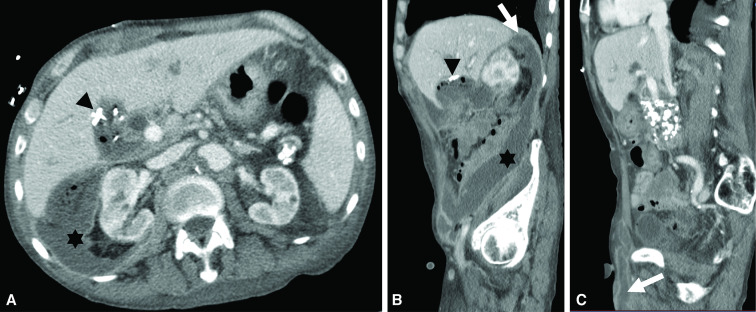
Abdominal CT post-cholecystectomy Day 8 showing extensive retroperitoneal multiloculated abscesses. Axial (A) and sagittal (B, C) images post-cholecystectomy with increasing leukocytosis. There is now extensive multiloculated right retroperitoneal abscess (asterisk, B) extending from the posterior liver margin at the level of the diaphragm (arrow, B) into the right lower quadrant anterior abdominal wall and scrotum (partially visualized, arrow, C) with contiguity with the gallbladder fossa that also contains fluid and gas along with surgical clips (arrowhead, A, B).
